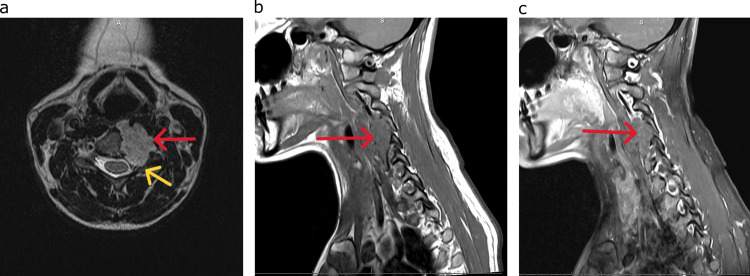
Pre-operative MRI scans (a) T2 axial view of the hyperintense lesion eroding the left C4 and C5 vertebral bodies (red arrow) with the compression of the left C4, C5 (yellow arrow), and C6 nerve roots. (b) T1 sagittal view of the pre-contrast image of the left-sided C4-C5 lesion (red arrow). (c) Sagittal post-contrast T1 image with mild enhancement of the left-sided C4-C5 lesion (red arrow). MRI: magnetic resonance imaging
